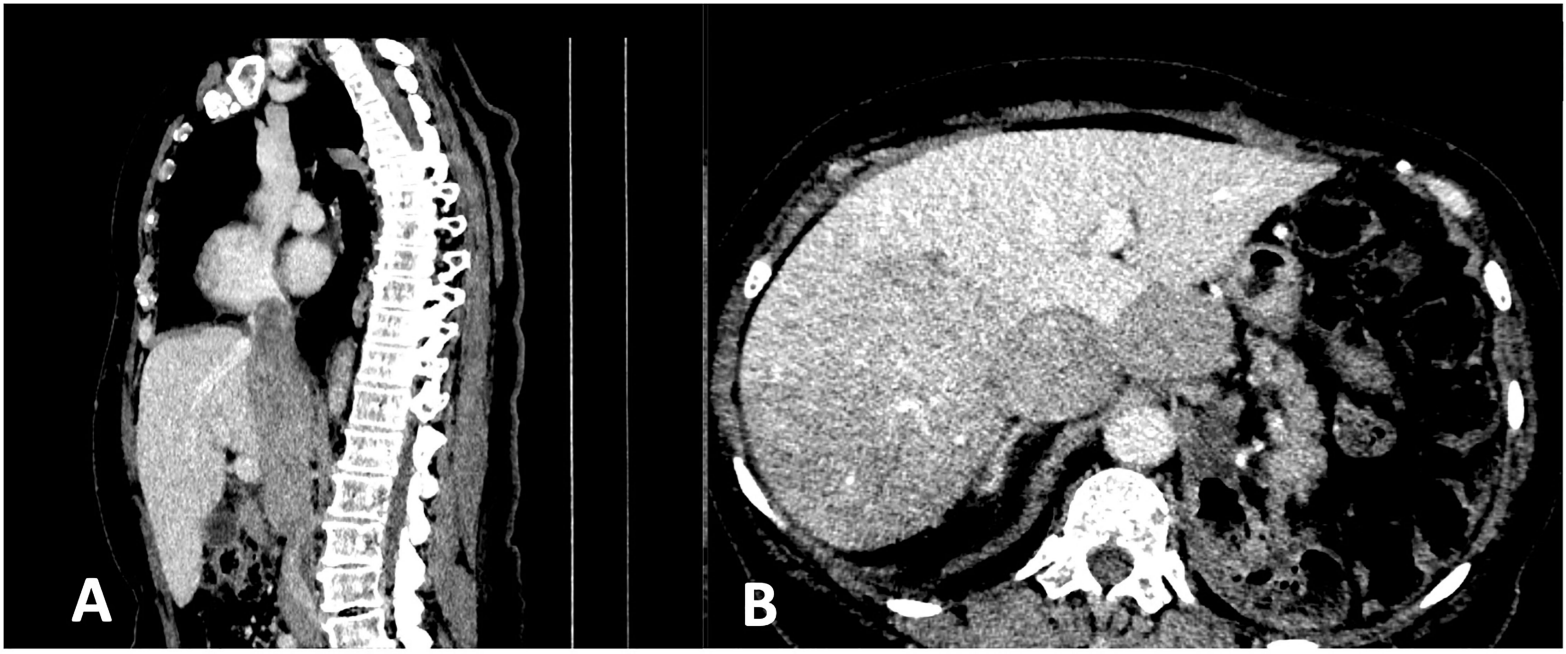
CT scan shows (A) the neoplastic thrombus almost completely obstructing inferior vena cava from L2/L3 to the right atrium; (B) perfusion changes in the liver.
